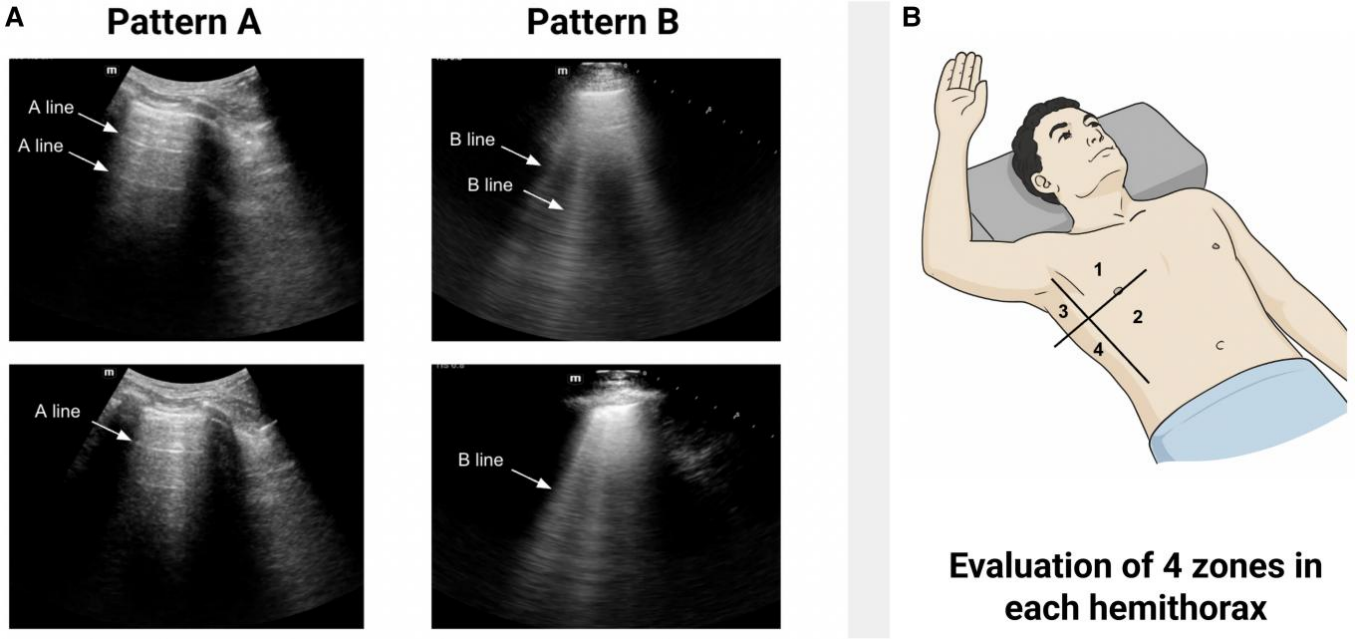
Lung ultrasound patterns and scanning protocol. (*A*) Pattern A shows normal lung aeration, with horizontal reverberation artefacts (A-lines) beneath a continuous pleural line. Pattern B shows interstitial syndrome, characterized by multiple vertical artefacts (B-lines) that arise from the pleura, reach the bottom of the screen, and erase A-lines. (*B*) The 8-zone lung ultrasound protocol involves the evaluation of four regions per hemithorax (two anterior and two lateral), as shown, with the patient in a supine or semi-recumbent position.
